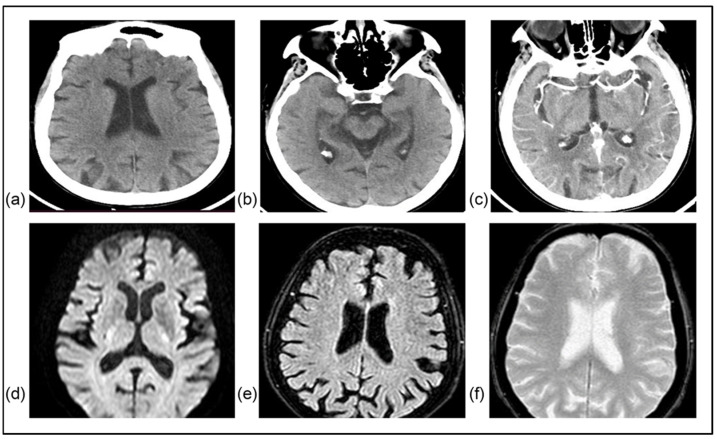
A 83-year-old woman with a medical history of hypertension, end-stage renal disease, and breast cancer in remission presented with right hemiparesis 3 h after symptom onset (NIHSS score: 6, mRS: 4). Initial NCCT (a,b) and CT angiography (CTA) (c) were unrevealing of parenchymal damage or large-vessel occlusion. She received IVT with alteplase within 30 min of arrival and experienced complete symptom resolution within the first 20 h. In MRI 9 days later, no signal hyperintensities were noted in DWI (d) and FLAIR (e) sequences. In T2* imaging (f), signal depression indicating bleeding or the presence of thrombus within a vessel was not identified. Thorough investigation during hospitalization did not reveal any likely cause of stroke mimic. This case represents an averted stroke: complete symptom resolution following reperfusion treatment, with negative subsequent imaging.
